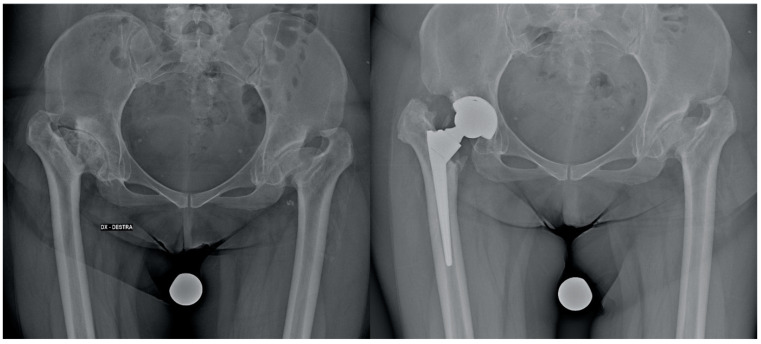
Pre-operative and post-operative X-rays of a patient treated with THA (conical stem) for hip dysplasia.
